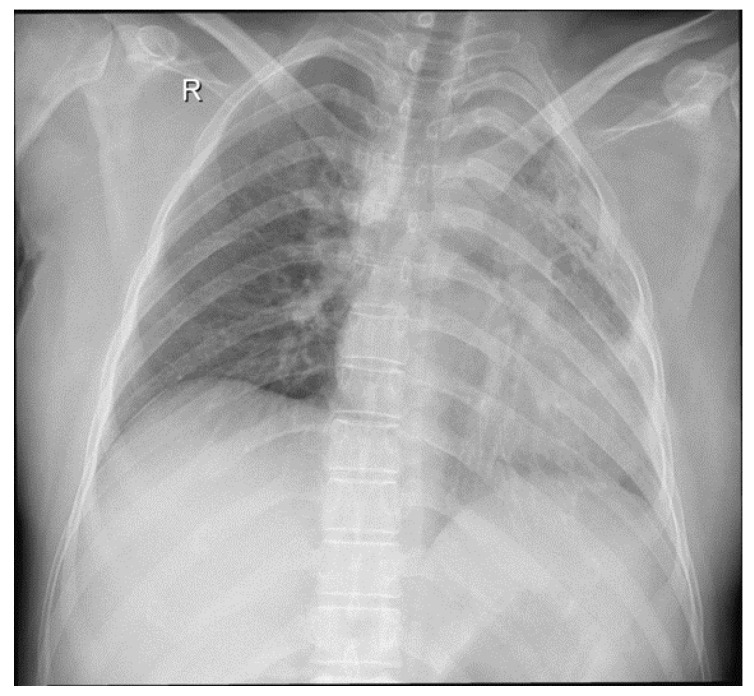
Chest radiograph: left pulmonary opacities, fibrosis, and bronchiectasis. Dense consolidation in the left upper lobe with clear evidence of volume loss.
